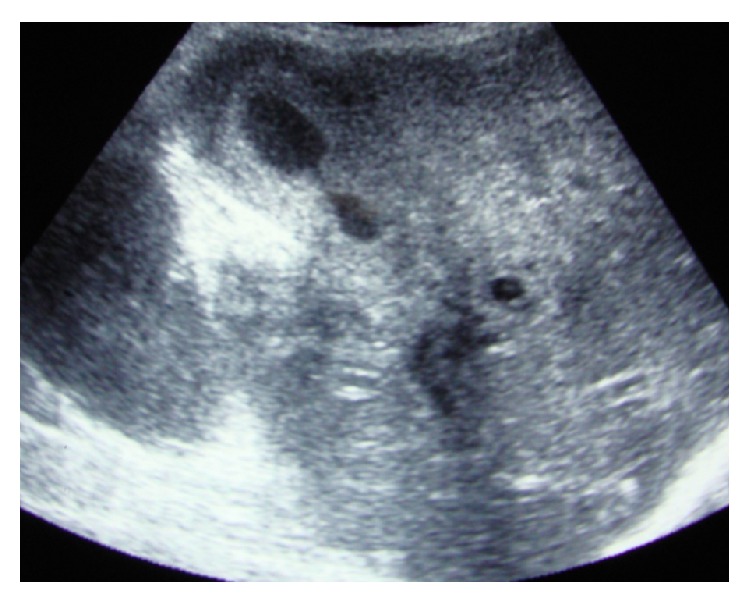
Ultrasound showing smooth uniform thickening of gallbladder wall.
